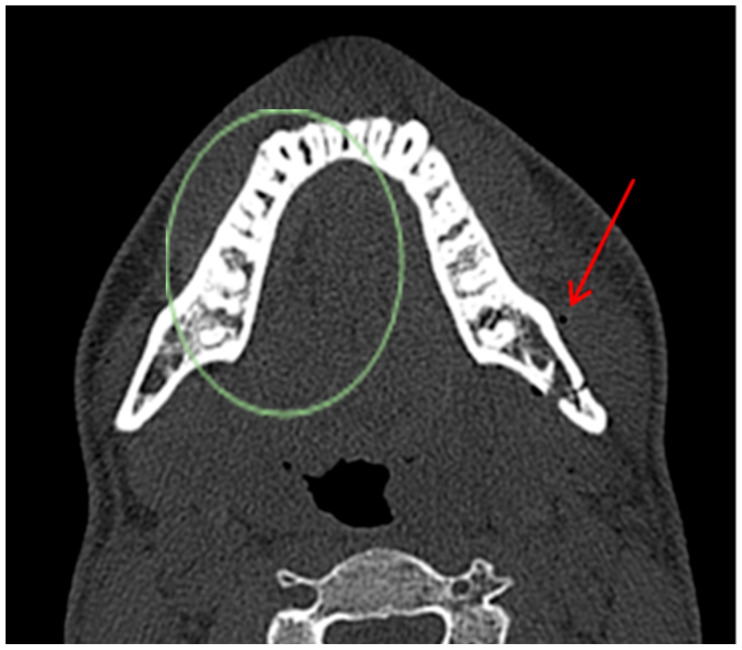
CT scan, horizontal view. A 22-year-old male patient diagnosed with a fracture of the left angle of the mandible with tooth 38 in the fracture line. The mechanism of injury was a hit to the chin, which could cause compound mandibular fractures. In this case, there was no combined fracture of the left angle + right body of the mandible. No fracture and AS were observed in the area of the body of the mandible on the right side, thus resulting in a true negative outcome (green circle). In this patient, a true positive result is also observed on the left side (red arrow).
